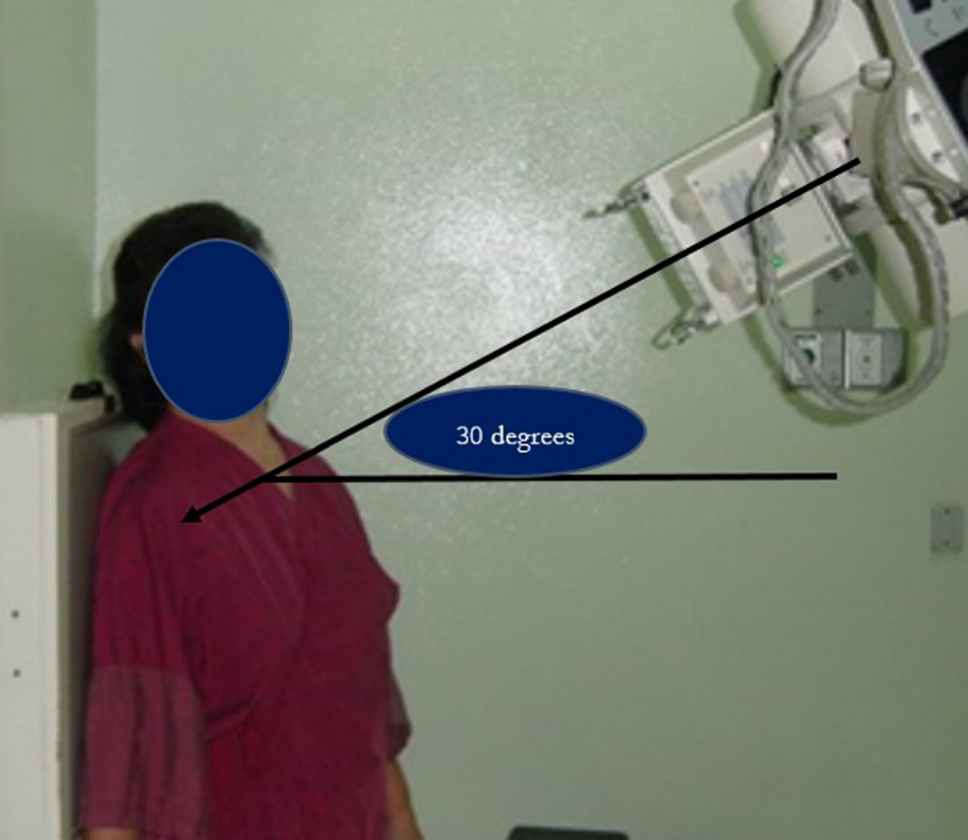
Standard position for the Rockwood tilt view film. The film was placed behind the shoulder of the patient, who was standing 35 cm between the cassette and the source. The X-ray beam was projected from the anterior direction at a 30° tilt from superior to inferior.
